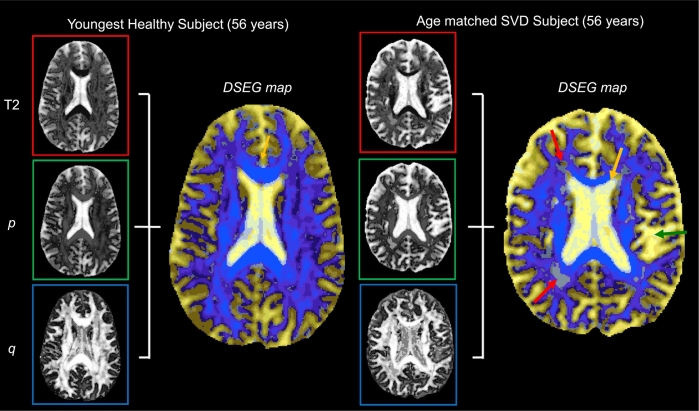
Two examples of how DSEG colour maps are generated using the scaled rank scores of signal from T2-weighted, p and q images. (a) Represents the DSEG map of the youngest healthy subject (56 years) used as the reference brain in DSEG *θ* calculations. (b) Represents age-matched subject with SVD (56 years). The orange arrow shows how the corpus callosum is represented by segment 8 while the red arrows highlight areas identified as WMH on FLAIR images represented by segments 15 and 16. The green arrow indicates greater volume of CSF space in the sulci in the SVD patient compared to the control. (For interpretation of the references to colour in this figure legend, the reader is referred to the web version of this article.)
